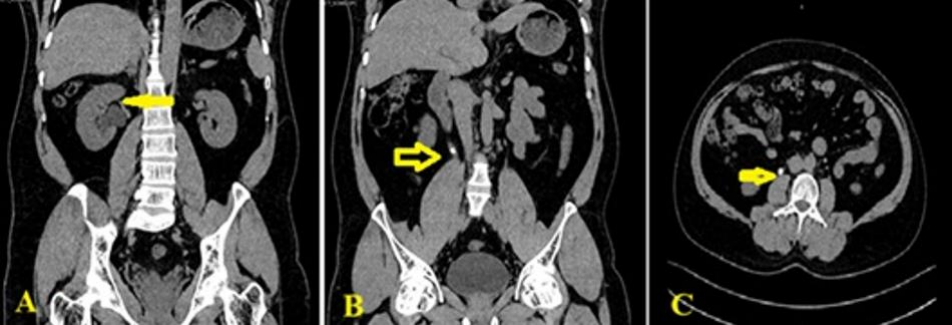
pre-procedural imaging of right ureteral obstruction: A) non-contrast axial Computed Tomography (CT) image of the abdomen demonstrating moderate hydronephrosis (arrow) secondary to an impacted ureteral calculus; B) axial CT image showing an 8 x 5mm hyperdense stone (arrow) at the right proximal ureter, adjacent to the lower border of the L3 vertebra; C) coronal reformatted CT image confirming the stone's location (arrow) with associated proximal ureteral dilation
